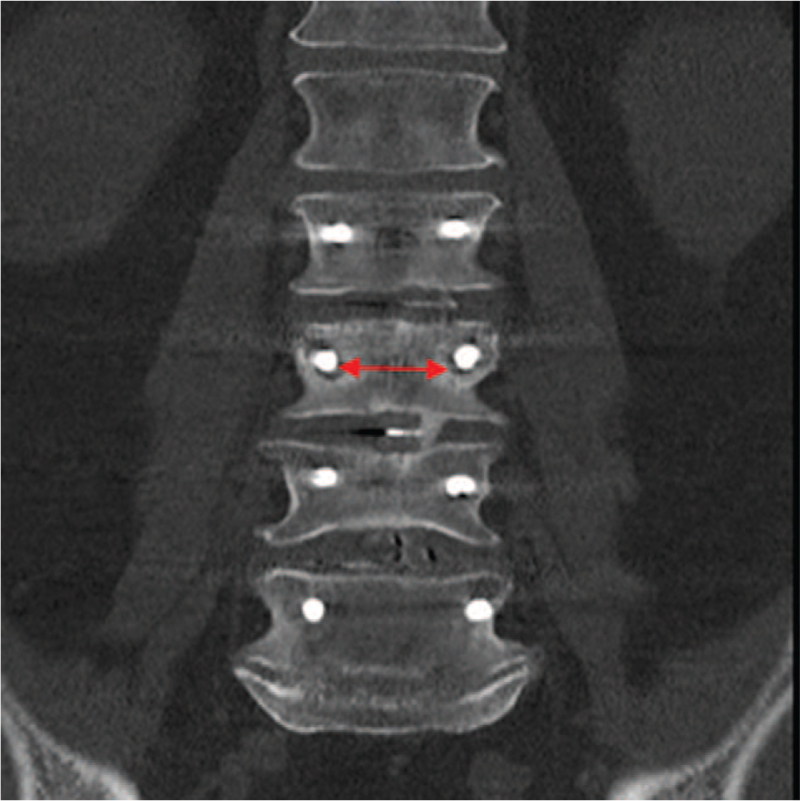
Coronary CT. Radiolucent zone without instability and with fusion of the segments L3-4. Red arrow showing radiolucent zones surrounding the pedicle screws of the lumbar spine L3 at the 12-month follow-up. Procedure in this case: no revision.
